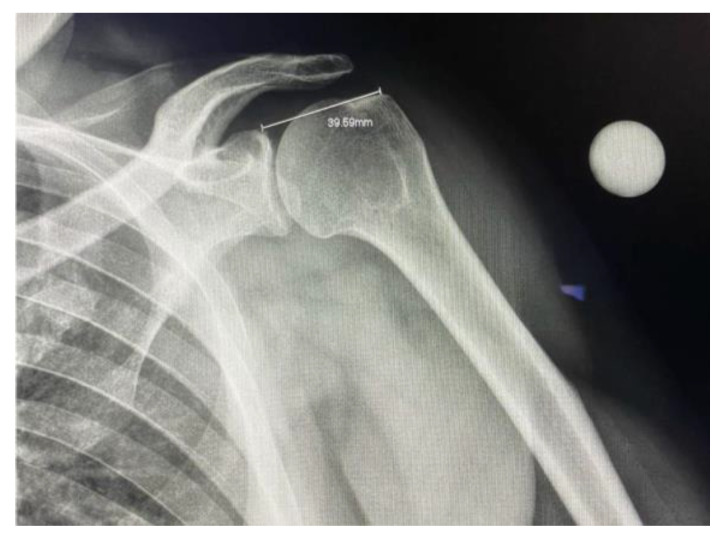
Plain film radiograph of the glenohumeral joint with a measurement of the distance between the superior glenoid and the insertion point of supraspinatus.
